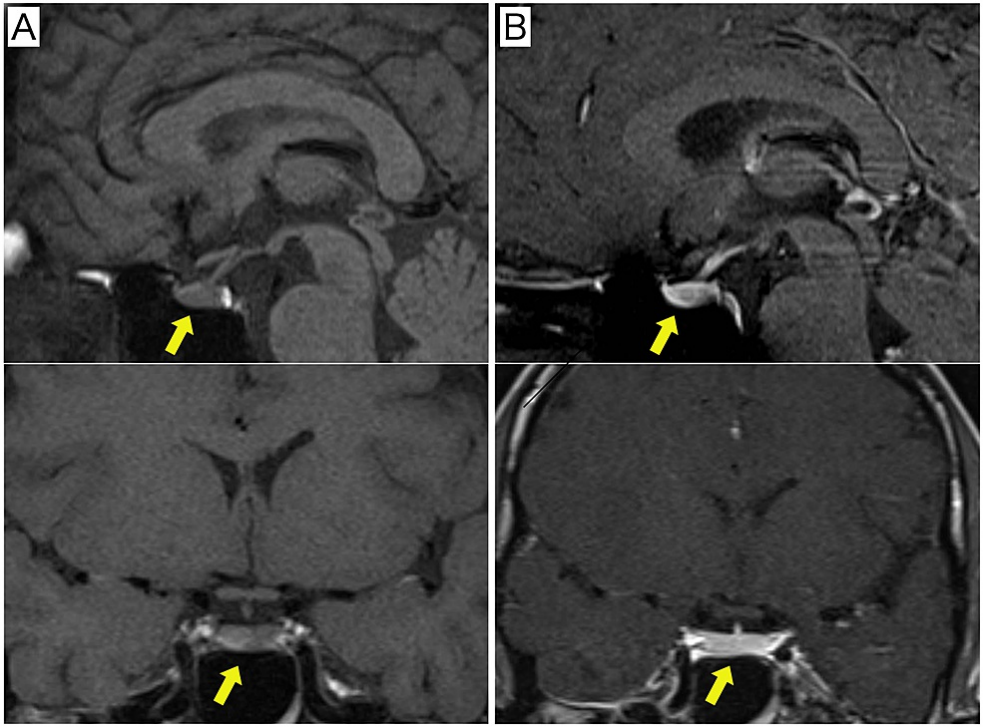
Magnetic resonance imaging of the hypothalamic–pituitary axis. Upper: sagittal plane. Lower: coronal plane. (A) T1-weighted image. (B) Gadolinium-enhanced T1-weighted image. Yellow arrows indicate the normal pituitary gland.
